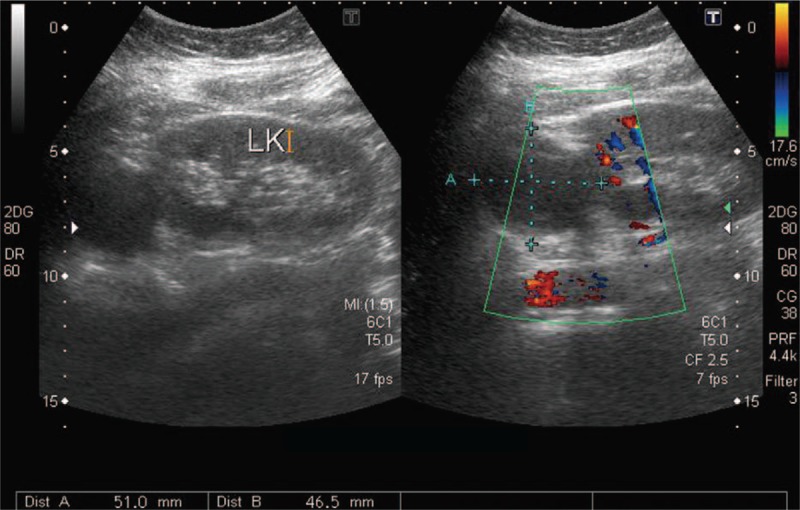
Ultrasound revealed a left renal cyst with the size of 5.1 × 4.7 cm.
